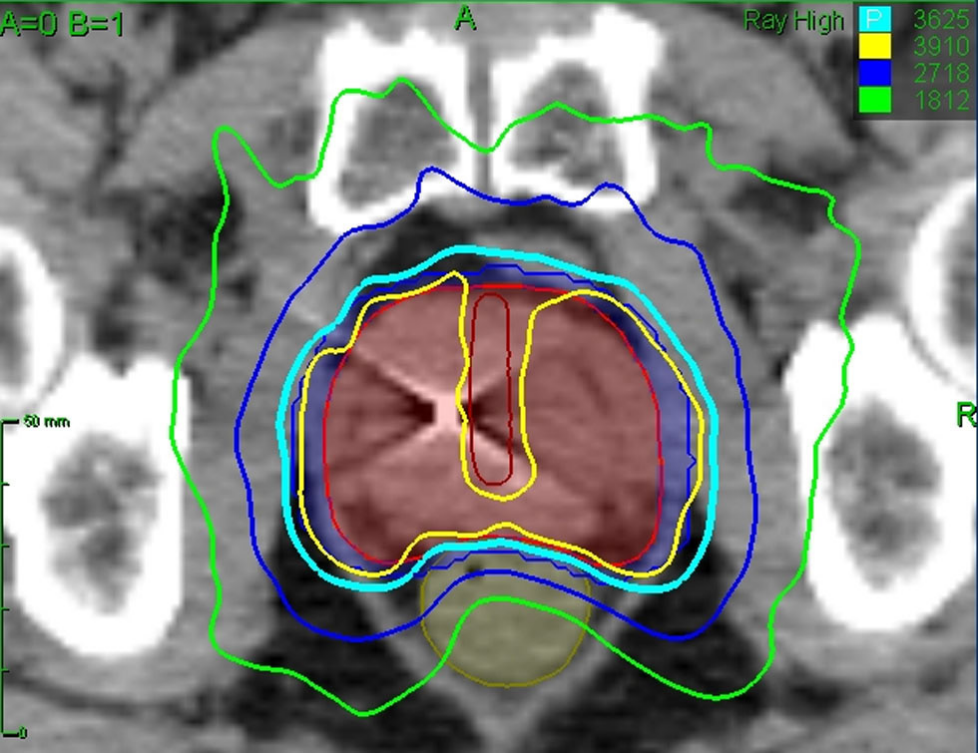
Treatment planning axial CT images demonstrating the prostatic urethra (dark red line), prostate (red line), and rectum (brown line). Isodose lines shown are as follows: 108% of the prescription dose (yellow line), 100% of the prescription dose (cyan line), 75% of the prescription dose (dark blue line), 50% of the prescription dose (green line).
